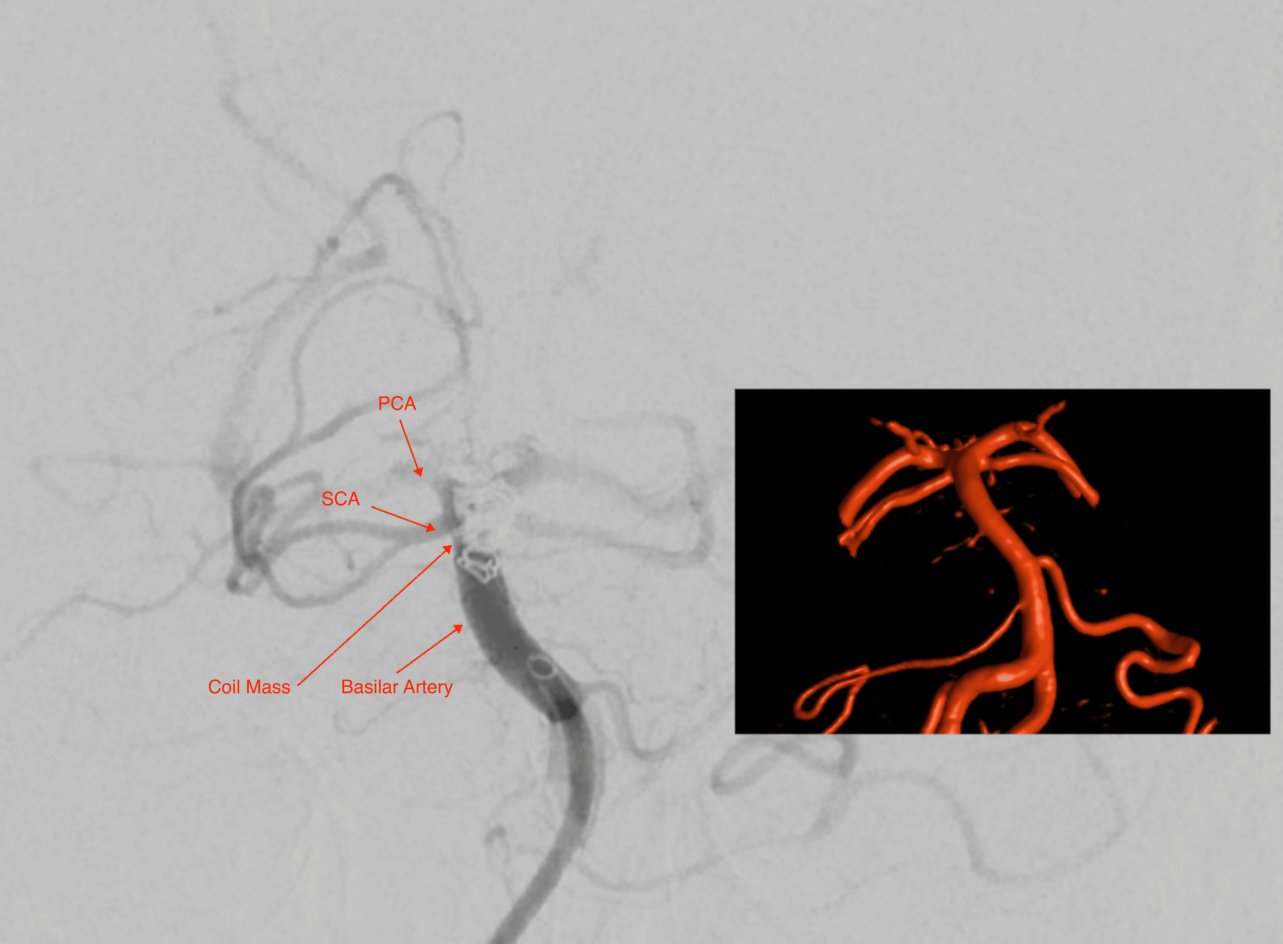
Intra-operative imaging of the final migrated coil mass position and its association with the basilar artery, superior cerebellar artery (SCA) and posterior cerebral artery (PCA). Overlayed 3-dimensional reconstructed CT helps visualise the anatomy.
